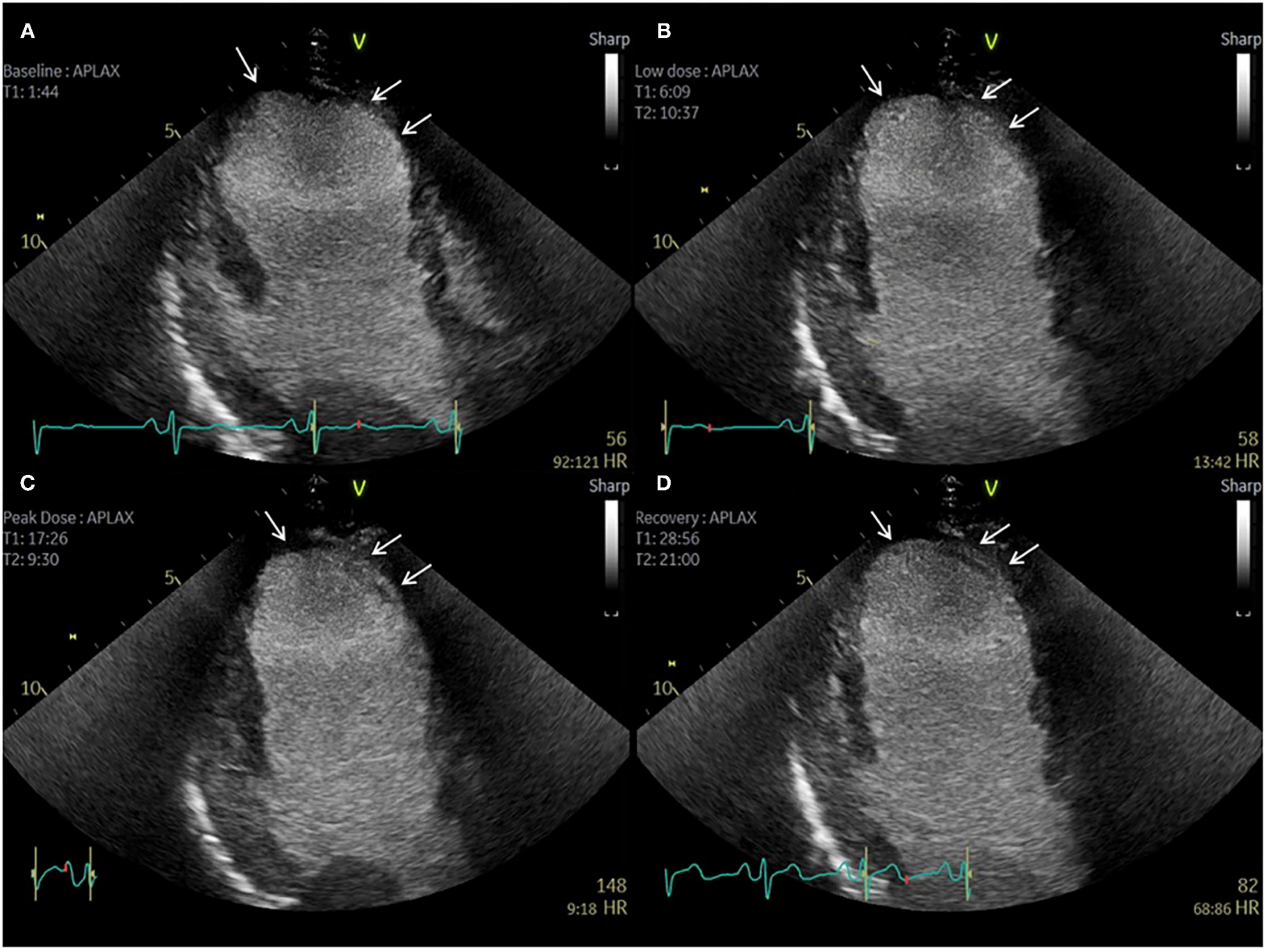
Contrast echocardiography post coronary artery bypass graft surgery. Sixty-three-year-old patient with previous CABG and atypical chest pain. (A–D) Apical 3 Chamber view. Baseline, low dose, peak dose, and recovery stages, respectively. Contrast Enhanced Images. Akinetic mid and apical antero-septal wall segments (arrow). No improvement in contractility of these segments during low dose stage confirms non-viable segments. Improvement in contractility of all other segments during low dose and peak dose suggests the presence of viable myocardium and no inducible ischaemia.
